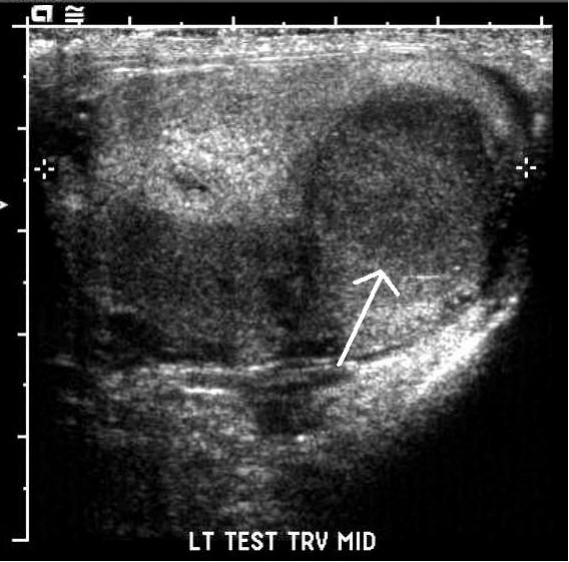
Ultrasound image of the left testicle. Arrow annotates abnormal mass lesion within testicular parenchyma.
